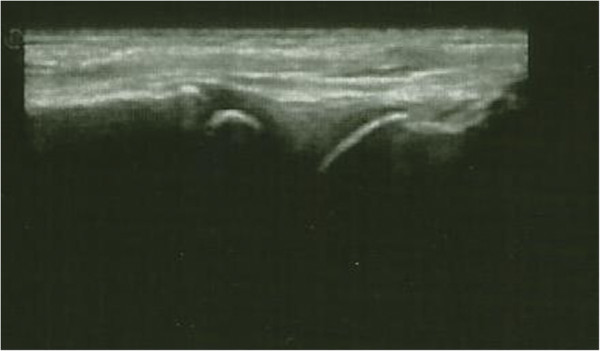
Case 1 left ankle ultrasound after treatment. Midsagittal12 − 5 MHz US image over the dorsal ankle showing normal joint recess, without hypoechoic fluid or synovial pannus.
